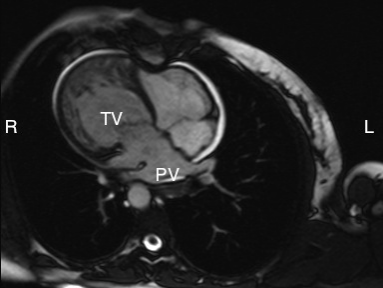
The magnetic resonance image shows a right-sided heart with the apex pointing to the right. The pulmonary veins are seen entering the left atrium (PV), with the tricuspid valve [TV] guarding the junction with the coarsely trabeculated systemic morphologically right ventricle.
